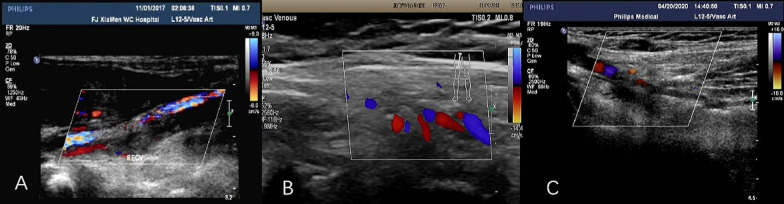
Ultrasound imaging of DVT. A Thrombus (Day 1) visualised in the right common femoral vein and superficial femoral vein of the right thigh. B (2 months later) Collateral circulation was formed. C (2 years 5 months later) Thrombus persisted in the right common femoral vein and superficial femoral vein
